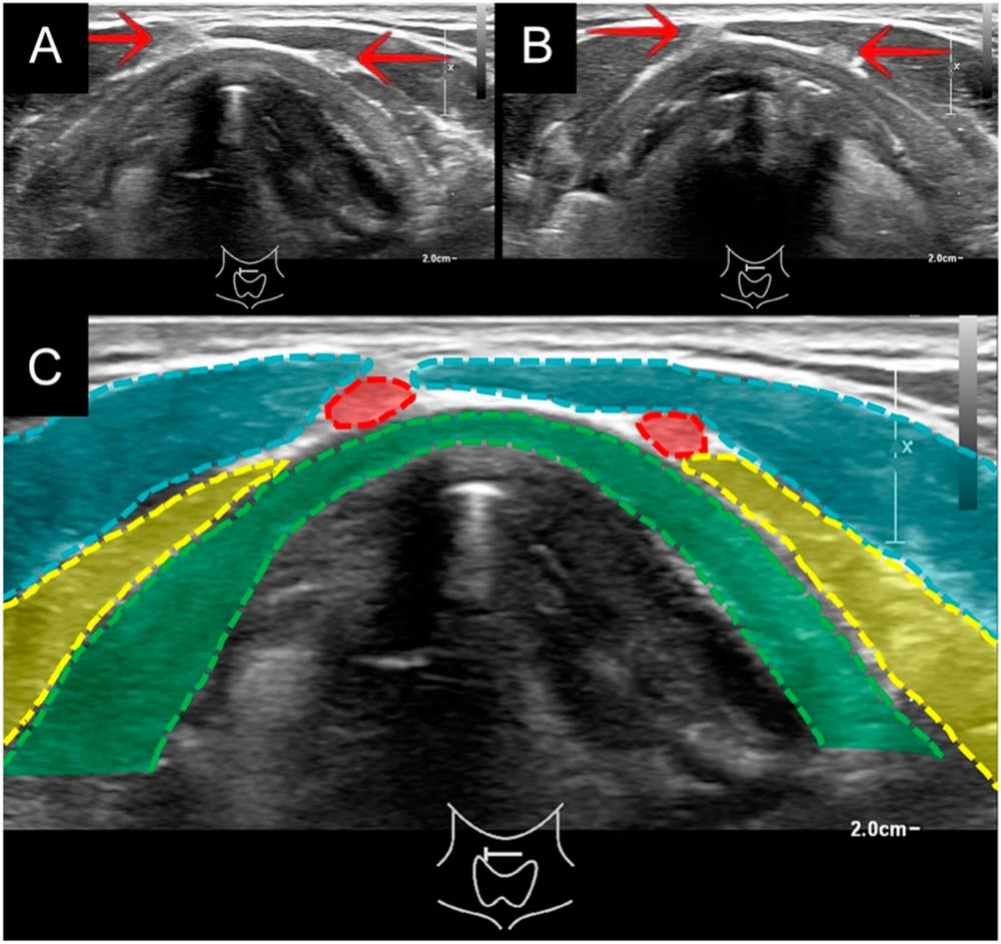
Transversal ultrasound images of the anterior neck. (A and B) The two pyramidal lobes (red arrows) are visible anterior to the thyroid cartilage at the level of the vocal cords (A) and slightly higher (B). (C) The anatomical structures shown in (A) are color-coded: two pyramidal lobes (red dashed lines), thyroid cartilage (green dashed lines), sternothyroid muscle (yellow dashed lines), and sternohyoid muscle (blue dashed lines).
